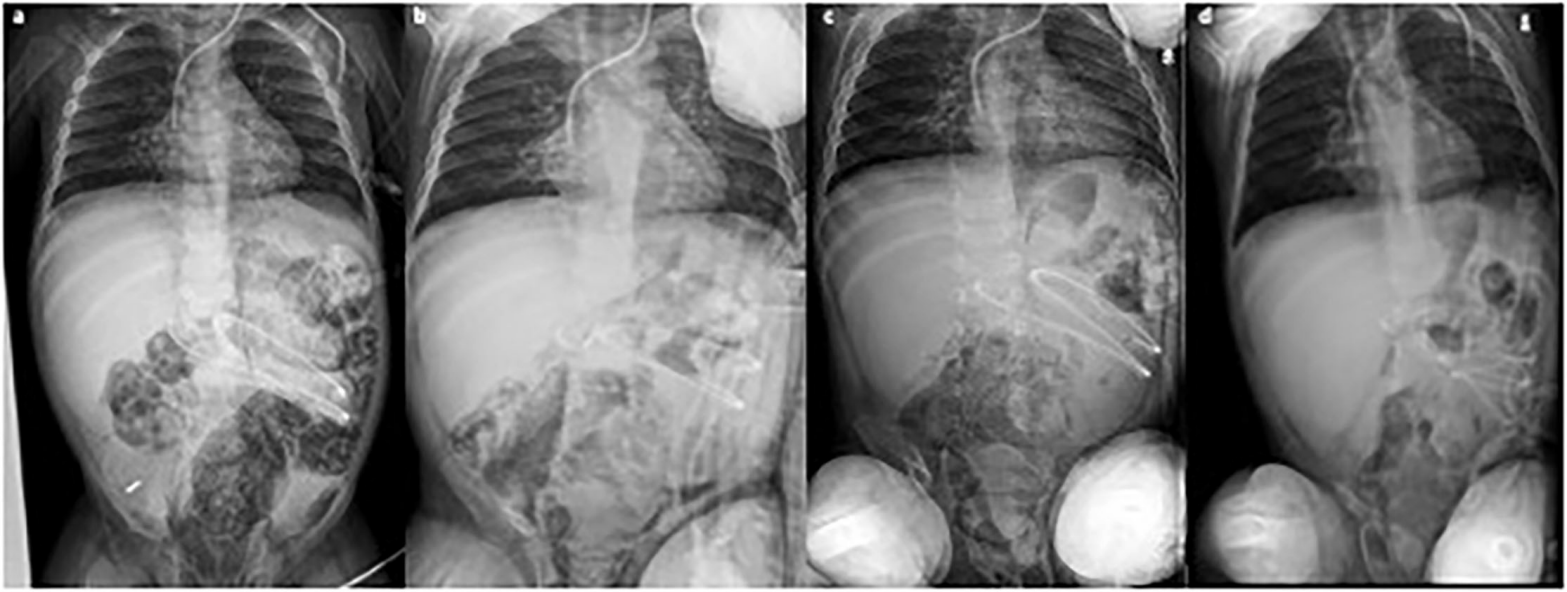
Consecutive abdominal x-rays [(a) day 1; (b) day 3; (c) day 5; (d) day 11] showing progressive reduction of intestinal pneumatosis. Clinical conditions remained fair during the whole period.
